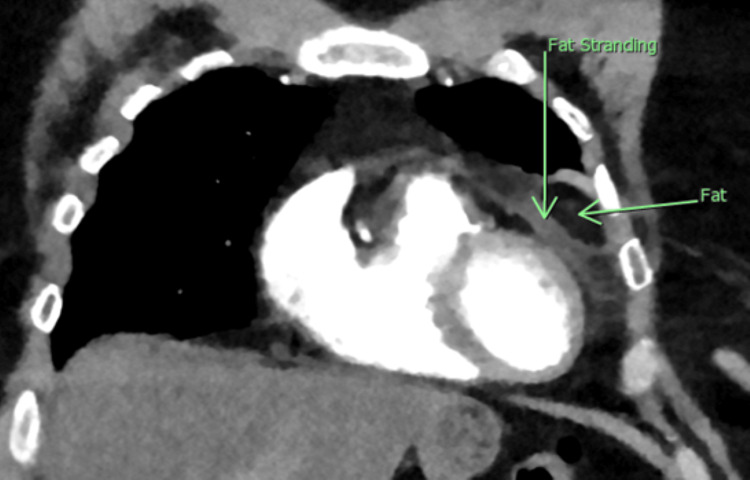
Coronal image from computed tomography showing a 4.2 cm x 1.8 cm x 3 cm lesion within the epicardial space using green arrows to differentiate the area of necrosis and surrounding fat stranding.
